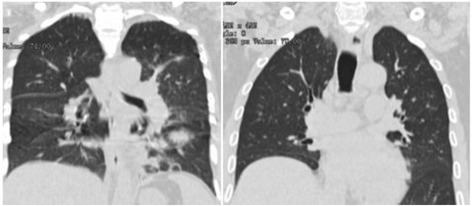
Computed Tomographic Scans of the Chest of case A and case B
